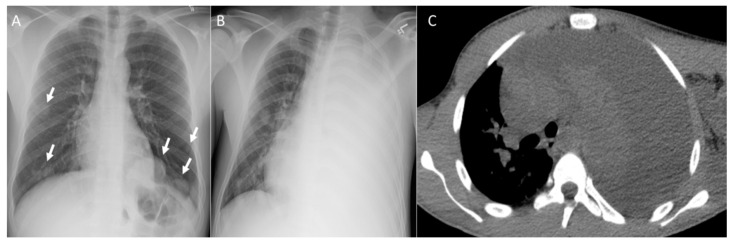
Patient with osteosarcoma with lung metastasis and malignant pleural effusion. Frontal chest radiograph (A) shows bilateral lung nodules and pleural-based left lower lobe masses (arrows). Frontal chest radiograph (B) and non-enhanced axial CT image (C) show an interval development of massive malignant left pleural effusion in a subsequent 3-week follow-up exam.
